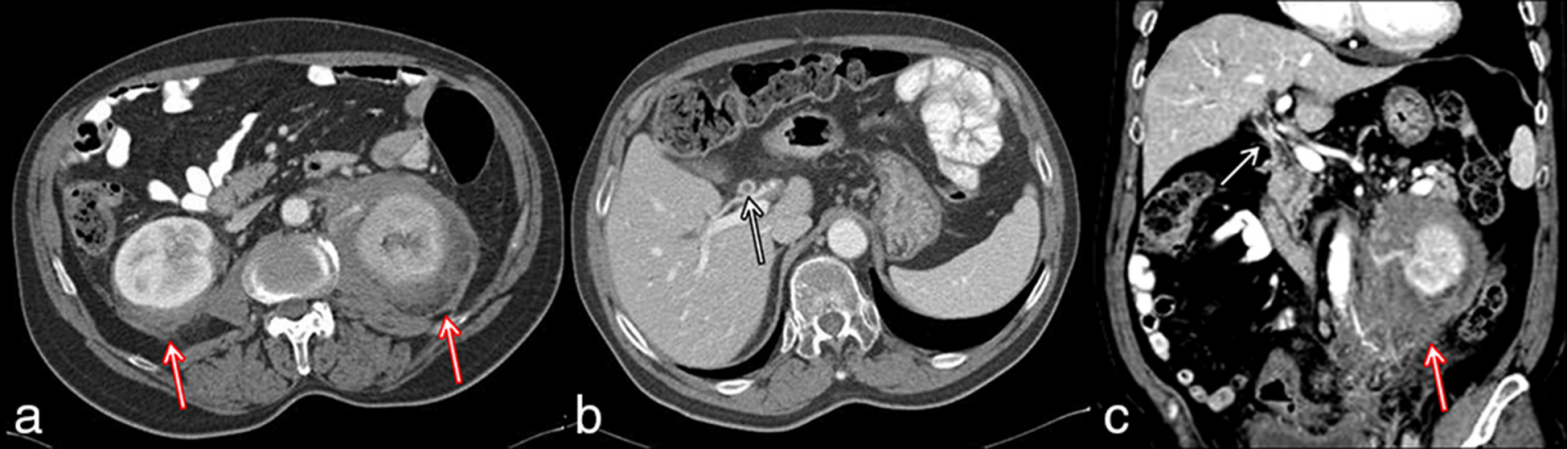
Axial portal-phase CT scan showing (a) a large amount of solid tissue in the left perirenal space and a smaller amount on the contralateral side (red arrows) and (b) a partial stenosis of the common bile duct owing to hypervascular eccentric tissue (white arrow). (c) Coronal portal-phase CT image showing left perirenal (red arrow) and peribiliary localization (white arrow).
